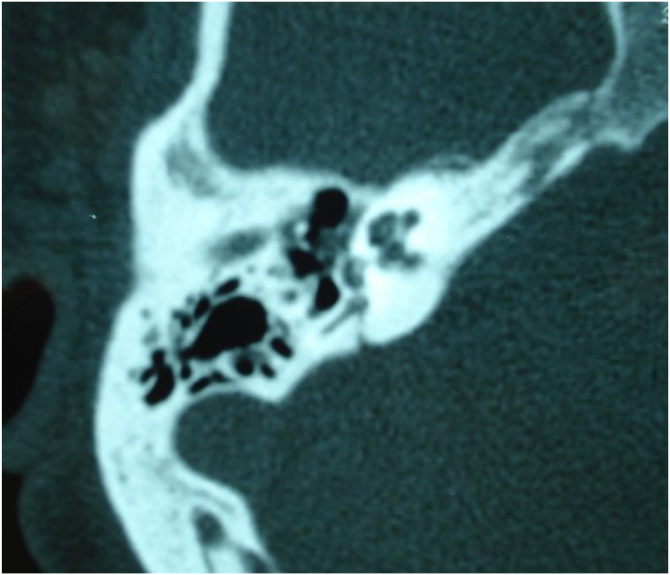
Computed tomography scan of the mastoid. Axial plane. Right ear. Patient with sensorineural hearing loss and peripheral facial paralysis. Presence of transverse fracture with injury to the labyrinth and basal turn that extends to the tympanic portion of the facial nerve.
